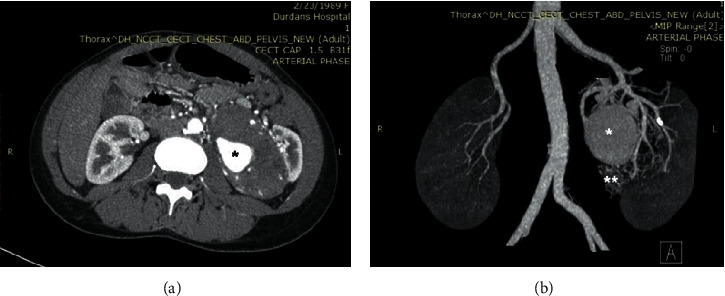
Contrast enhanced CT images of the tumour. (a) An axial section showing the left renal tumour showing the aneurysm (asterisk). The tumour involves the entire kidney except a thin rim of normally enhancing renal tissue at the upper outer region. (b) CT renal angiogram showing multiple feeding arteries and the aneurysm (asterisk). The vasculature of the extrarenal cystic nodule (double asterisks) is also seen inferior to the aneurysm.
